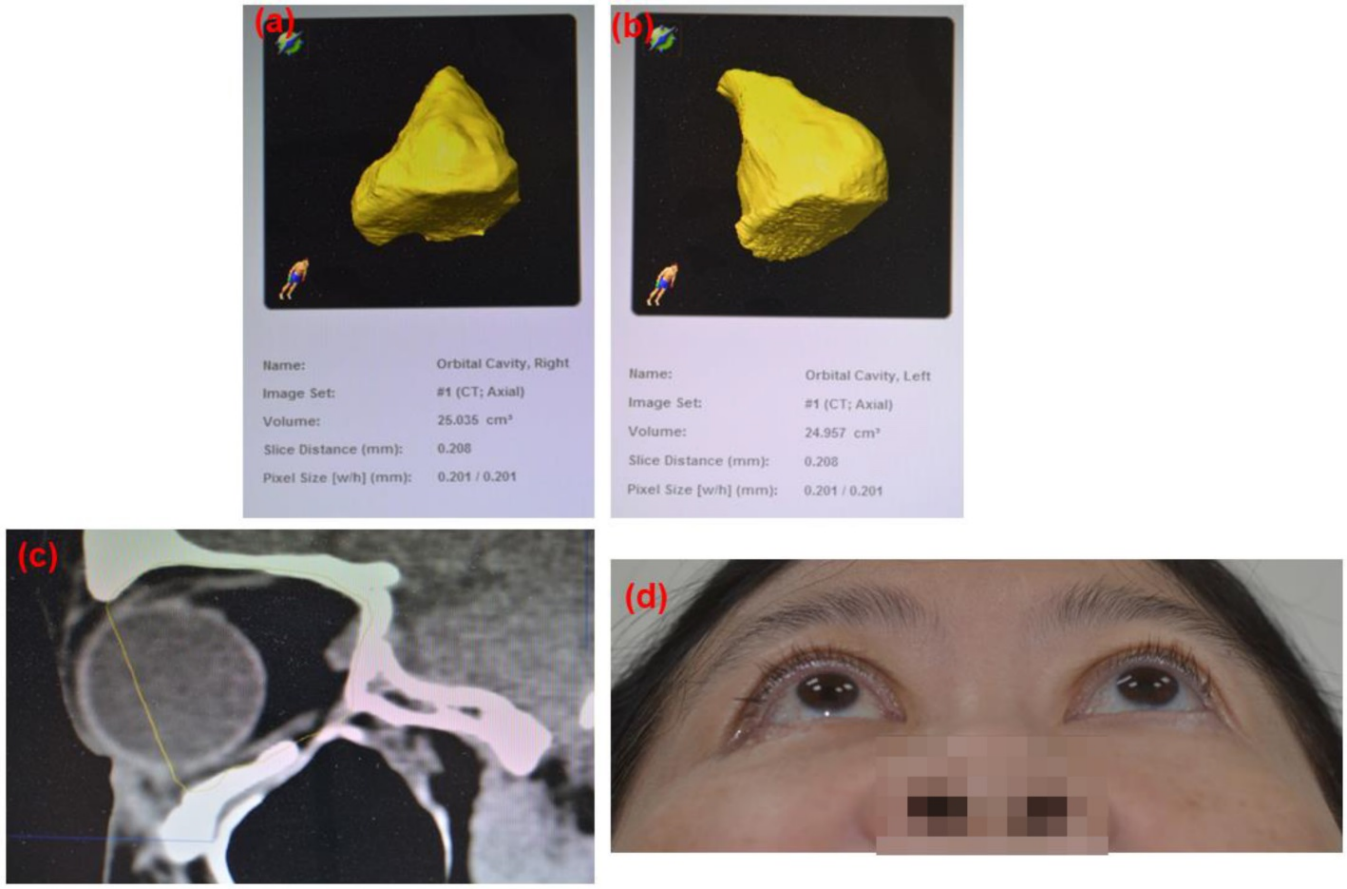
Post-operation 8 weeks follow up CT images based on the 3D reconstruction of the orbital cavity by Brainlab® software of Case 10 (Rt side Zygomatic complex fracture). (a) Rt side orbital volume: 25.035 cm^3^, (b) Lt side orbital volume: 24.957 cm^3^ Difference: 0.078 cm^3^ (c) sagittal view. ABCcolla^®^ Collagen Bone graft was implanted (d) post-operation 2 months follow-up. Without any complaint was noted.
